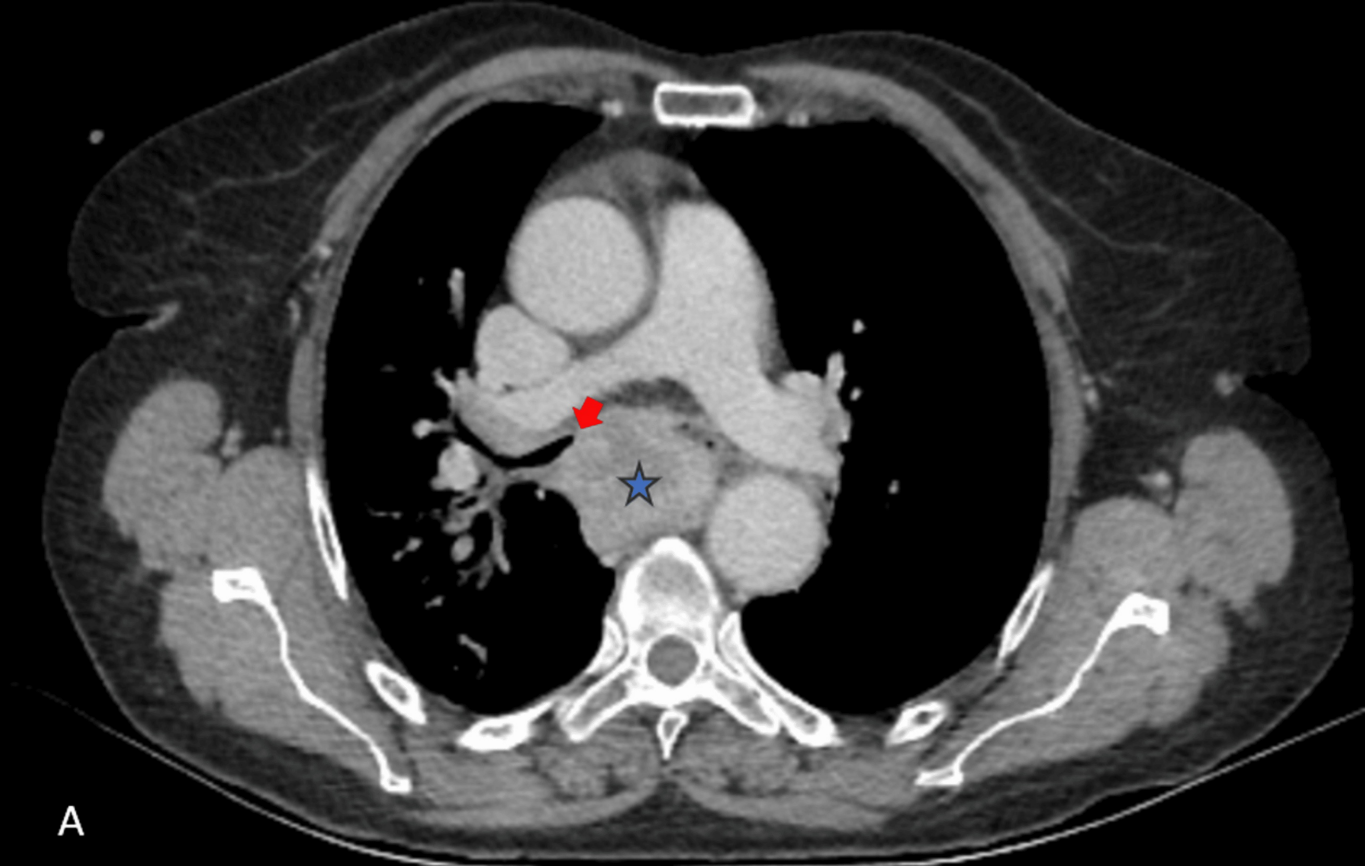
Axial contrast-enhanced CT showing a subcarinal mediastinal mass (blue star) invading the carina (red arrow).
